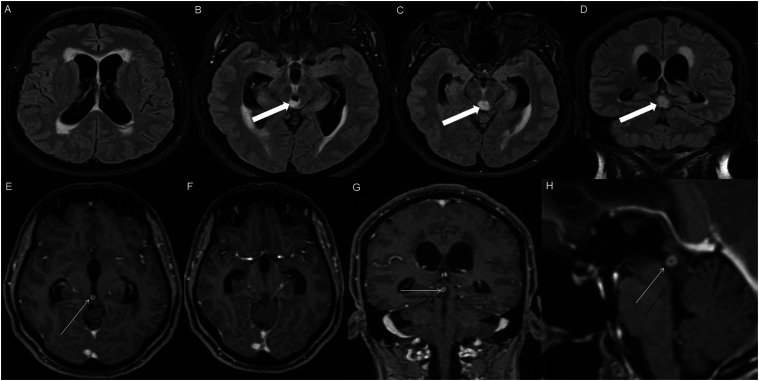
Axial FLAIR (A-C), coronal FLAIR (D), axial post-contrast T1 weighted images (E-F), coronal post-contrast T1 weighted image (G) and sagittal post-contrast T1 weighted image (H), show a FLAIR hyperintense mass involving the quadrigeminal plate and periaqueductal area (B-D, thick white arrows), with mixed non-enhancing (F-H, thin black arrow) and ring-enhancement components (E,G-H, thin white arrow). There is mass effect and obstructive hydrocephalus, with transependymal CSF flow.
